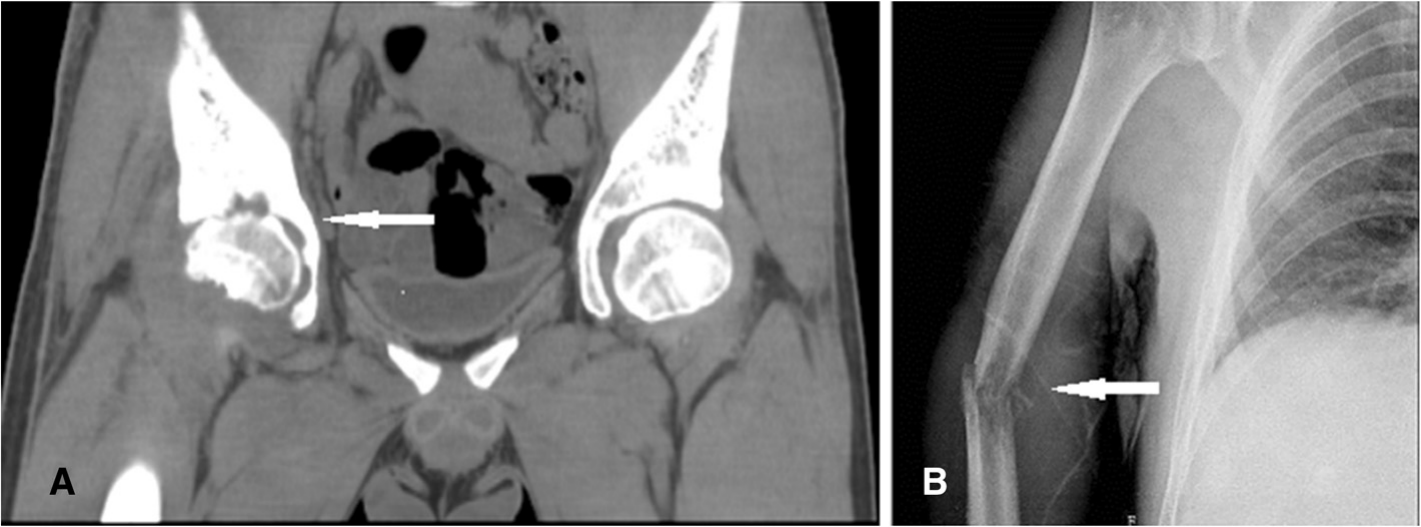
Radiography and computed tomography showing periosteal proliferation in the innominatum and femur (A), and a pathologic fracture (B) in the humerus.
